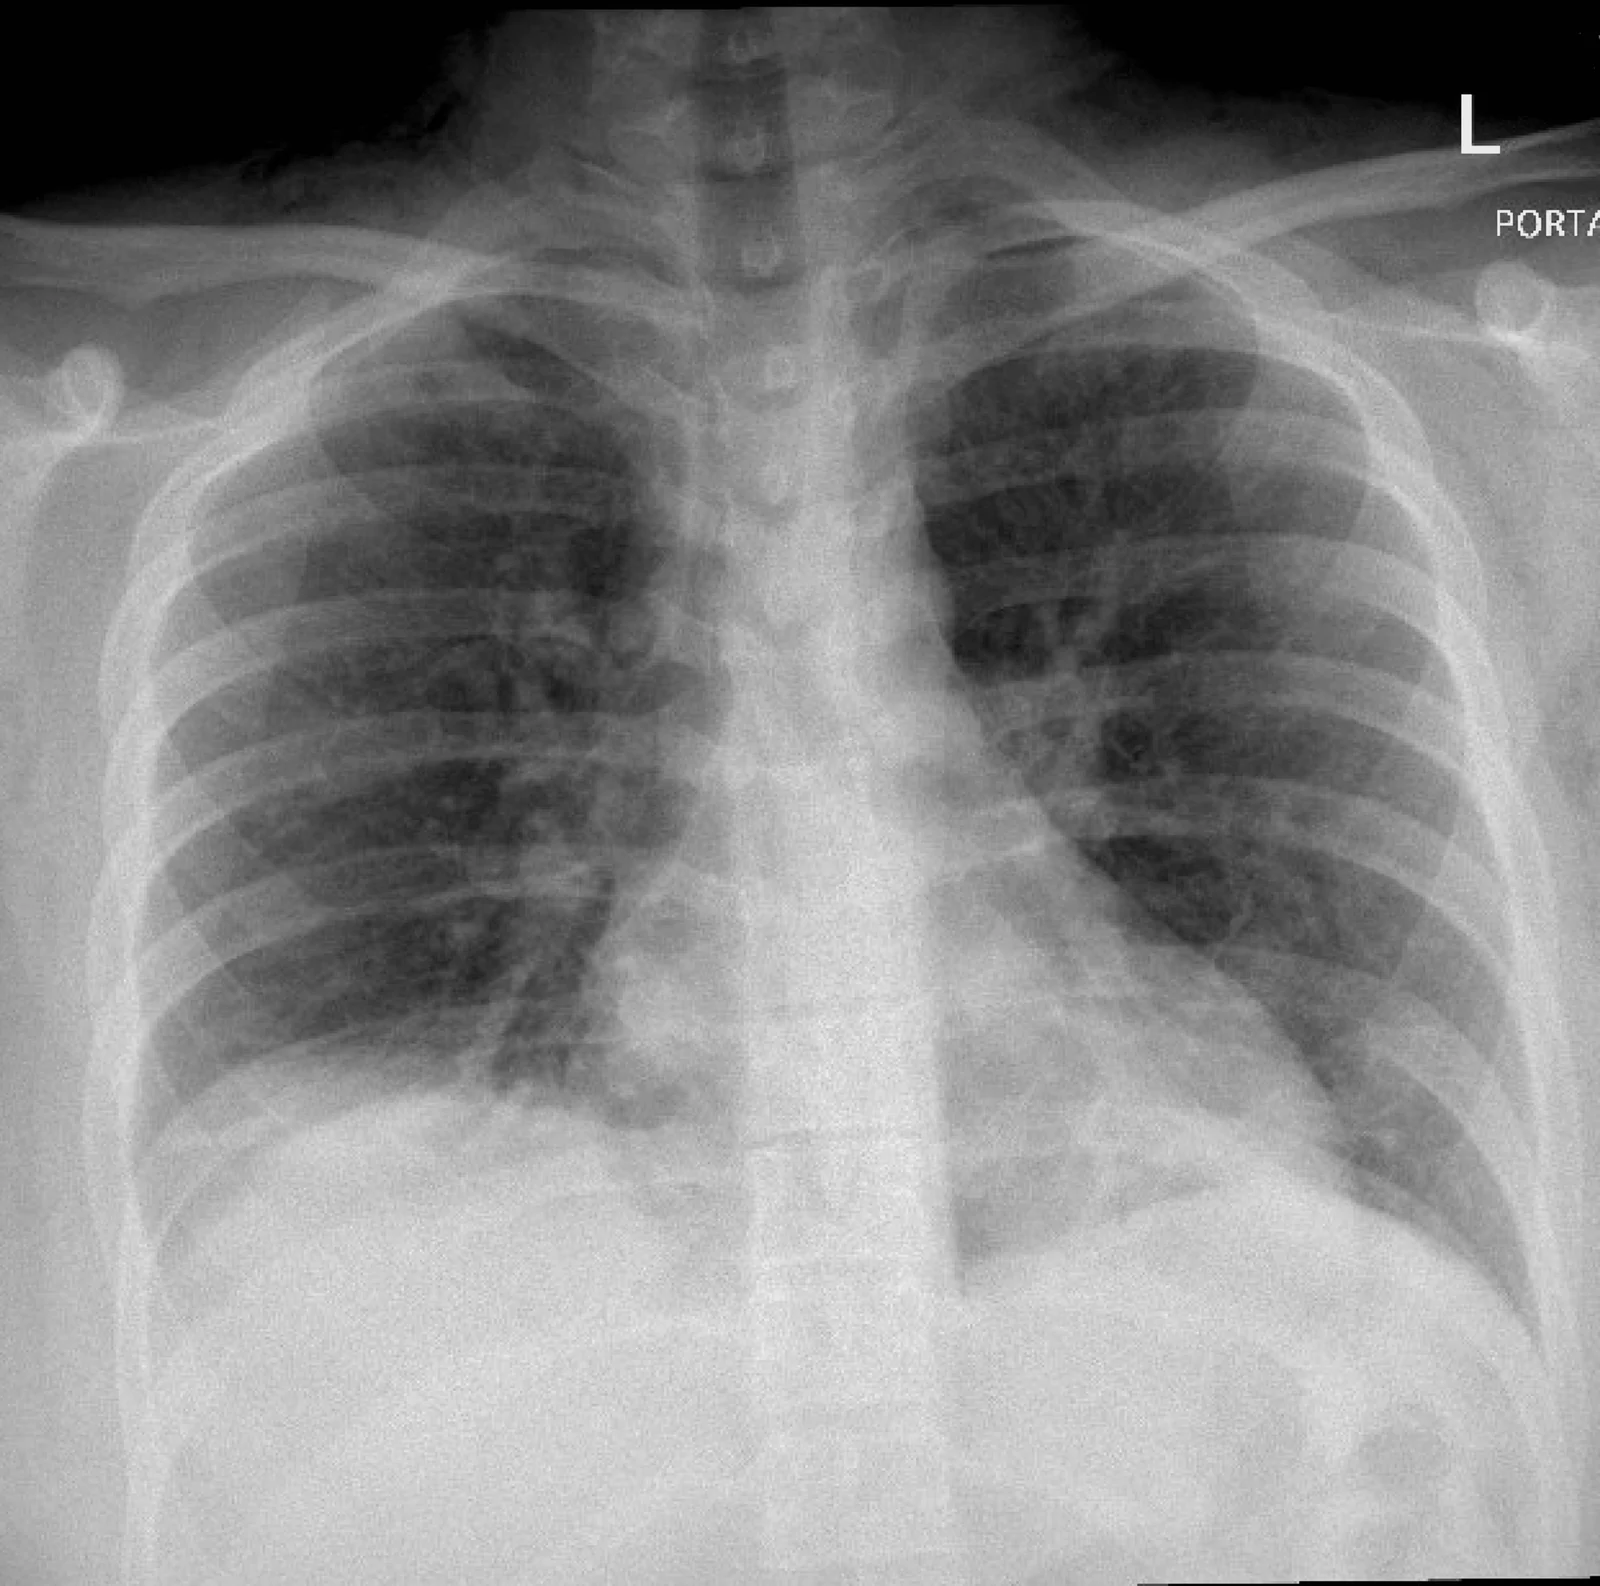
Chest X-ray demonstrating interval improvement of the pneumomediastinum, with persistent residual right paratracheal pneumomediastinum and pneumopericardium.
